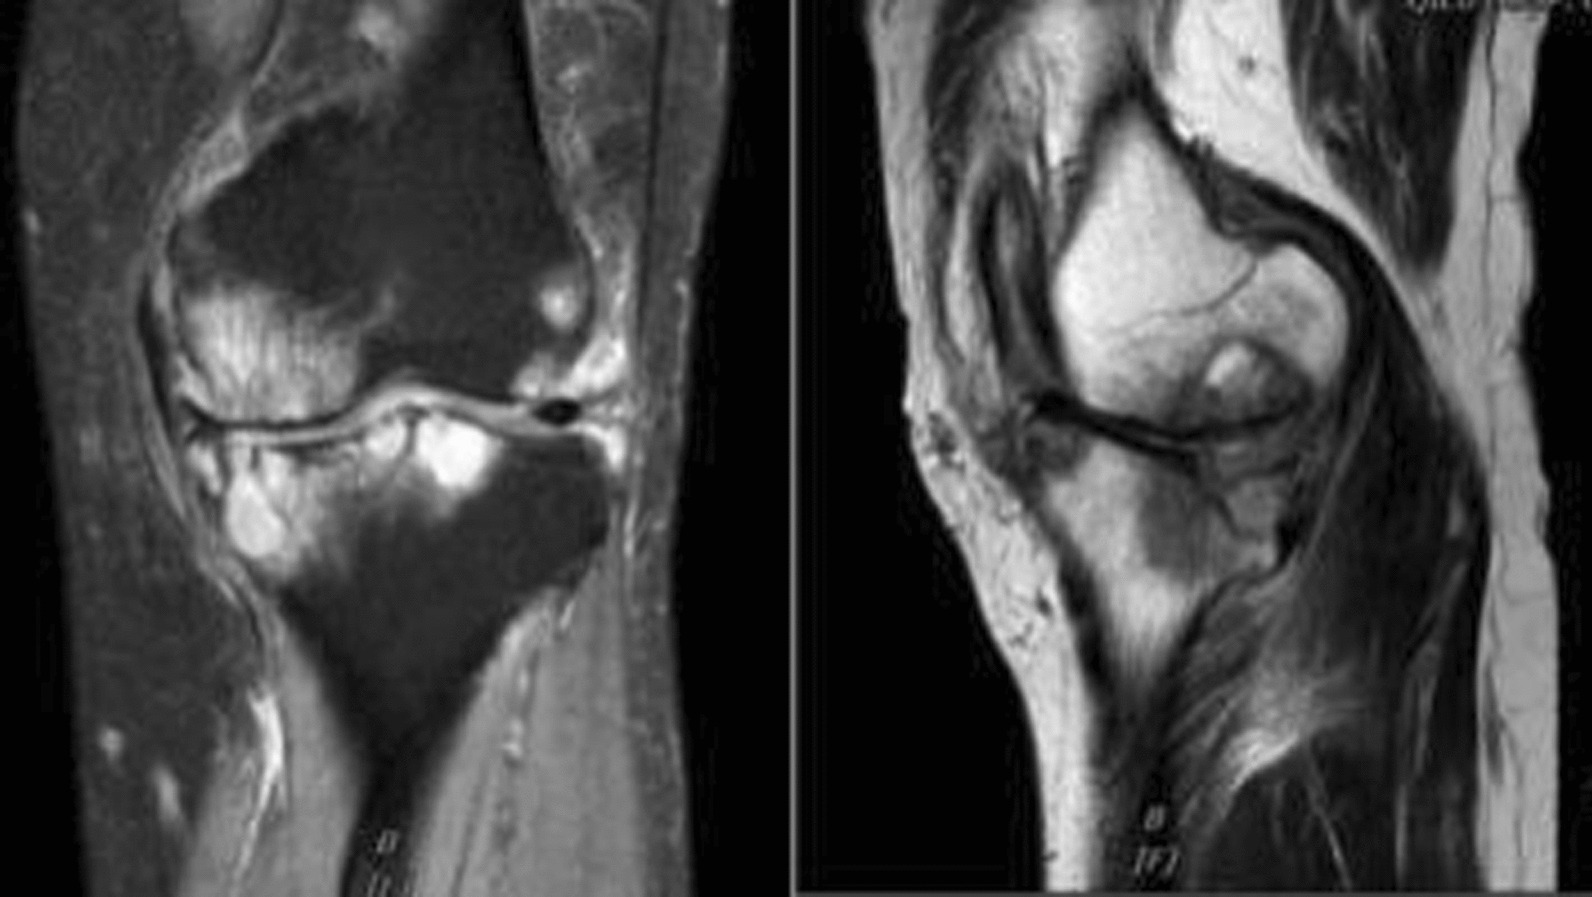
Magnetic resonance imaging (MRI) of the left knee showing multiple cystic changes in the distal femur and proximal tibia, bone marrow edema, and no inflammatory changes in the soft tissues surrounding the knee joint
